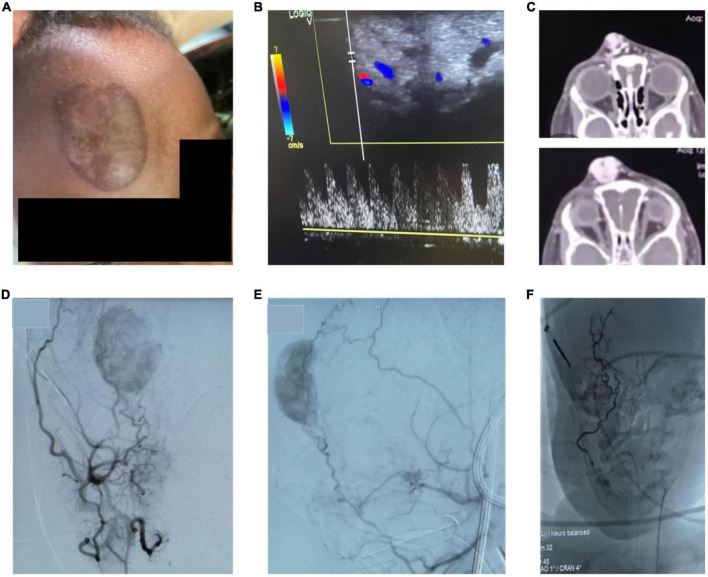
(A,B) Pre-procedural picture of a clinical examination showing a pulsatile, warm AVM of the forehead with corresponding high flow on doppler ultrasound. (C) CT-images with contrast show a highly vascularized lesion with arterial feeders and venous drainage via the ophthalmic veins. (D,E) Angiographic images of the AVM by contrast administration through the external carotid artery confirm the facial artery as the major feeding vessel. Further, the frontal branch of the temporal artery has small feeding branches. (F) After embolization of the main feeding vessels with liquid embolic material occluding the arterial vascular network, the intravascular cast formed by the liquid embolic agent can be appreciated. The patient underwent subsequent surgical resection of the lesion.
